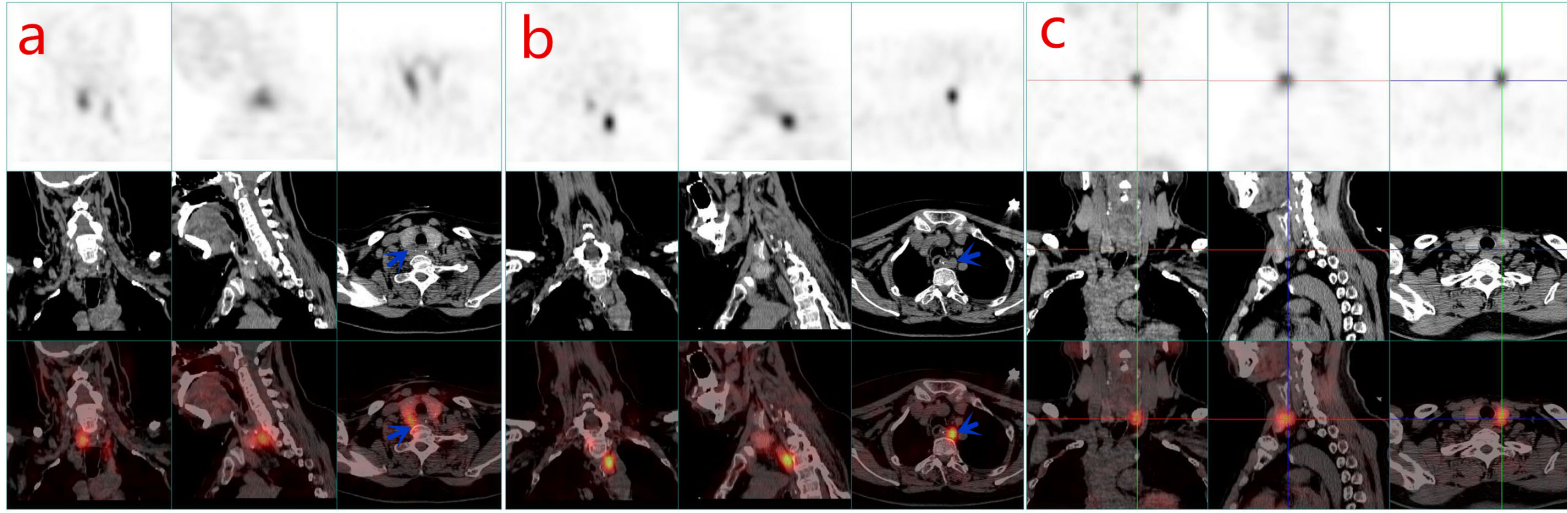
Functional nuclear medicine imaging with single photon emission CT (SPECT) combined with anatomical X-CT (SPECT/CT) has improved the sensitivity and specificity for many clinical applications. 99mTc-MIBI SPECT/CT is the first-line imaging modality for detecting parathyroid tumors in PHPT. Local tomography and image fusion techniques significantly improve the positive rate of detection. The first row is SPECT image, the second row is X-ray CT image, and the third row is SPECT and CT fused image obtained by the hybrid system. 99mTc-MIBI SPECT/CT: In the right parathyroid bed region (a) and the upper mediastinum (b), two lesions with high 99mTc-MIBI uptake were clearly shown in the transverse, coronal and sagittal planes (blue arrow). *In situ* and ectopic parathyroid adenomas were confirmed by postoperative pathology. PET/CT is the second-line imaging modality for detecting parathyroid tumors in PHPT. The first row is PET image, the second row is X-ray CT image, and the third row is PET and CT fused image obtained by the hybrid system. 18F-FDG PET/CT: In the left parathyroid gland bed region (c), a high 18F-fluorodeoxyglucose metabolic lesion was clearly displayed in the transverse, coronal and sagittal planes. Parathyroid adenoma was confirmed by postoperative pathology.
